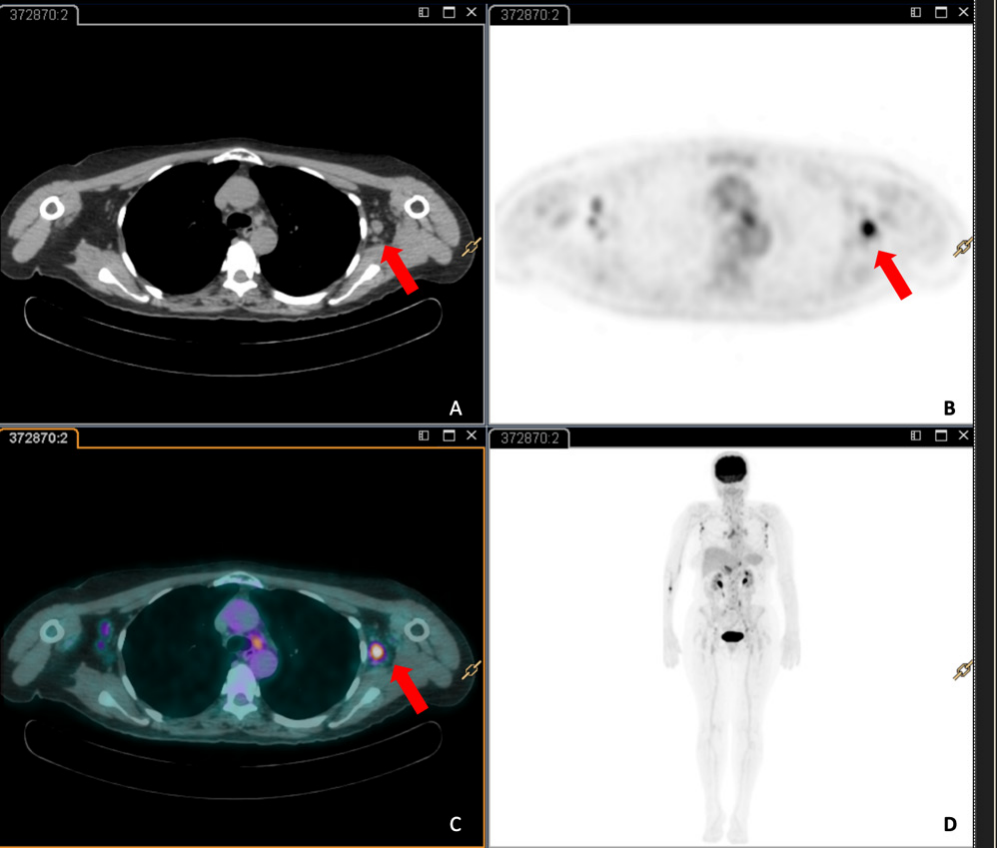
Axial view of total body CT (A), PET imaging (B), the hybrid PET/CT (c) and coronal view of total body PET (D). The images (A) shows an enlarged node in the left axilla (red aarow) which demonstrates abnormal metabolic activity (B, C) (red arrows). PET, positron emission tomography.
